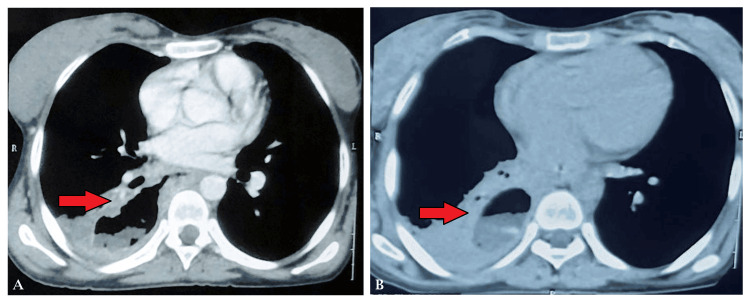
(A) An axial section of the CT of the thorax showing a communication between the esophagus and the right bronchus. (B) An axial section of the CT of the thorax showing a fluid-filled cavity in the right posterior segment of the lower lobe
